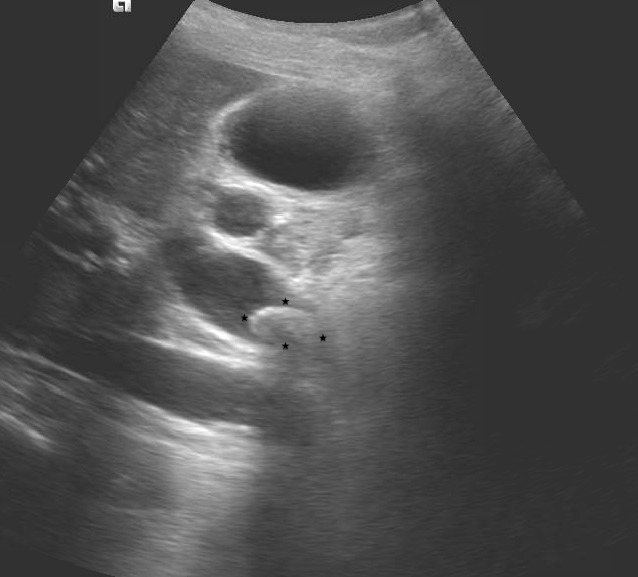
Ultrasound showing significant proximal common bile duct dilatation measuring 2.1 cm A stone within the proximal to mid-common bile duct measuring 8.9 mm in short axis by 15.1 mm in long axis is visualized.
